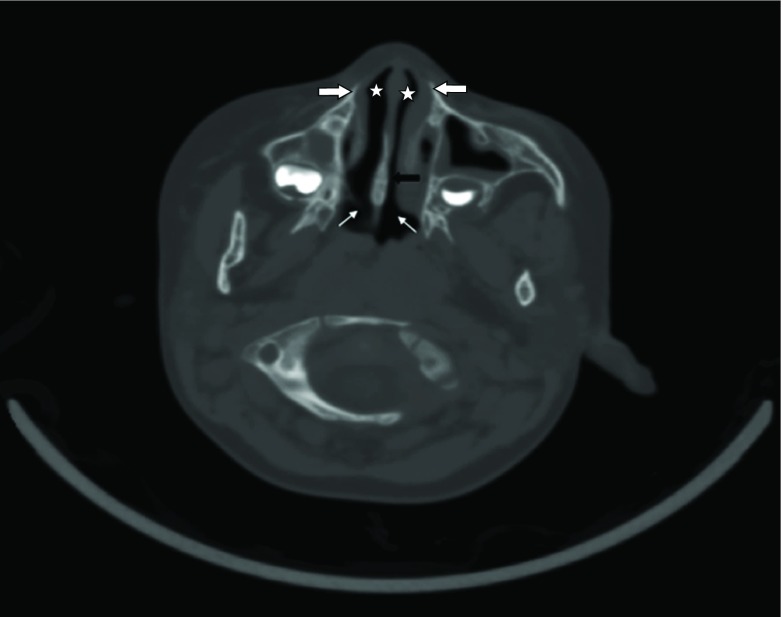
Transverse CT image obtained through the nasal cavity in a normal child. Thick white arrows indicate normal nasal processes. Note the calibre of the normal pyriform apertures (stars). Thick black arrow indicates nasal septum. Thin white arrows indicate posterior choanae.
